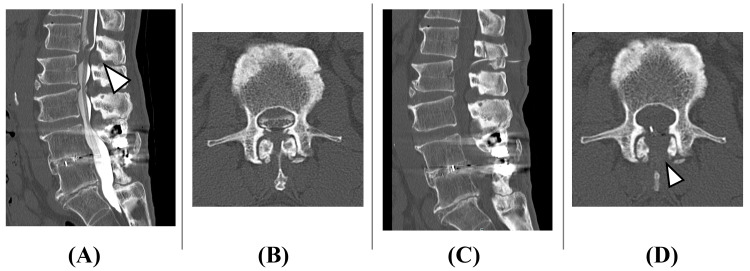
Lumbar CT images Preoperative lumbar sagittal section (A) and preoperative lumbar axial section (B) are CT images after myelography, showing severe spinal canal stenosis at the L1/2 level (white arrowhead in panel A). Postoperative lumbar sagittal section (C) and postoperative lumbar axial section (D) show that the spinous process has been split longitudinally and spinal canal decompression has been achieved. Despite the relatively narrow laminar width, both facet joints are preserved, and trumpet-shaped laminectomy has been performed (white arrowhead in panel D).
